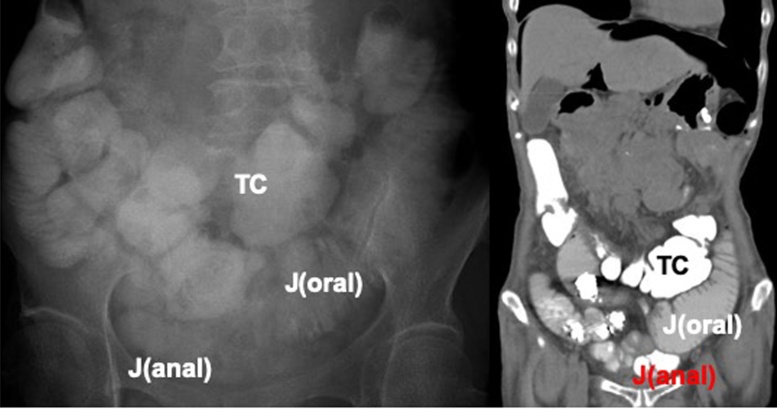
Radiological images after jejunojejunostomy (a) Gastrograffin passing from the jejunal loop (J(oral)) into the jejunum anal to the anastomosis (J(anal)). Contrast material given 2 days prior was seen in the transverse colon (TC). (b) CT scan taken 4 h after gastrograffin injestion.
